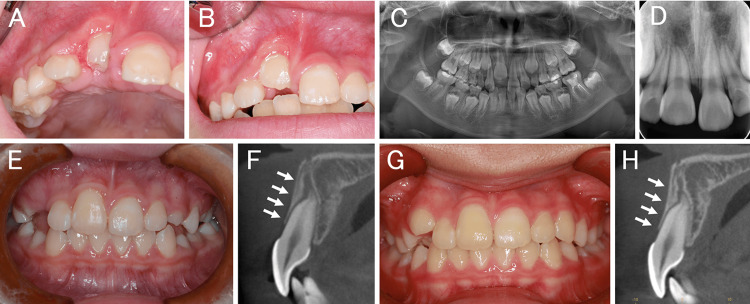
Treatment process (A) Intraoral photograph one week after surgery. (B,C) Intraoral photograph and panorama radiographic one month after surgery. (D) Dental radiographic four months after surgery. (E) Intraoral photograph and (F) cone-beam computed tomography nine months after surgery. The white arrow line indicates the position of the horizontal level. (G) Intraoral photograph and (H) cone-beam computed tomography one year and three months after surgery. The white arrow line indicates the position of the horizontal level.
